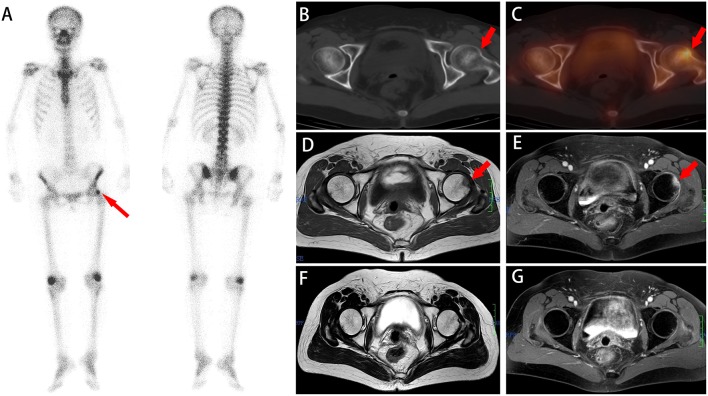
A whole-body scan (A) revealed a focal area of high ^99m^Tc-MDP activity in the left femur (red arrow). No additional areas of abnormal ^99m^Tc-MDP uptake were identified in the remainder of the skeleton. Axial (B,C) CT and SPECT/ CT imaging showed focally increased ^99m^Tc-MDP uptake without density changes in the proximal part of the femur. Axial (D,E) MR images show high signal on T1WI and T2WI that disappeared 5 months later (F,G). The diagnosis of IF was established based on a combined assessment of clinical and radiologic follow-up.
